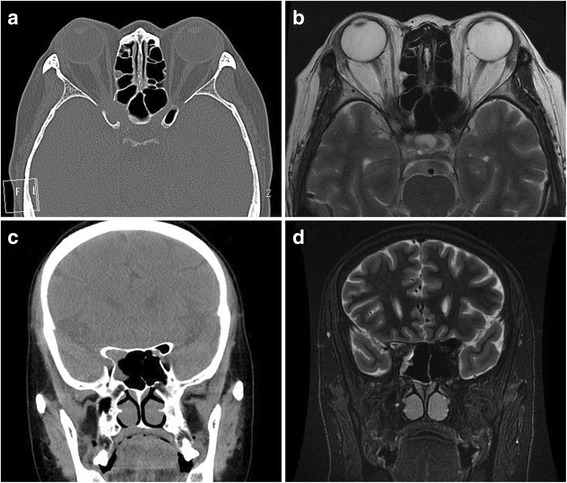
Neuroimaging for case 3, female, 56y, invasive fungal sphenoid sinusitis (right, VA = no light perception). a: Axial CT scan with expansion and opacification of the right optic canal and optic nerve, which was invaded by fungal organisms. b: Axial, T2-weighted MRI scan showing hypointense signal primarily adjacent to the right optic canal. c: Coronal CT scan showing opacification of the right anterior clinoid process. d: Coronal, T2-weighted, contrast-enhanced MRI scan with abnormal signal in the area of the right orbital apex
